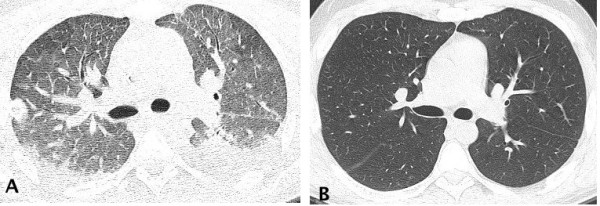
A 36-year-old male patient with pulmonary toxoplasmosis. Axial view of chest CT scan in A displays bilateral ground glass opacities and smooth septal and peribronchovascular thickening. In figure B, a complete resolution of the findings 3 months after treatment was evident.’
